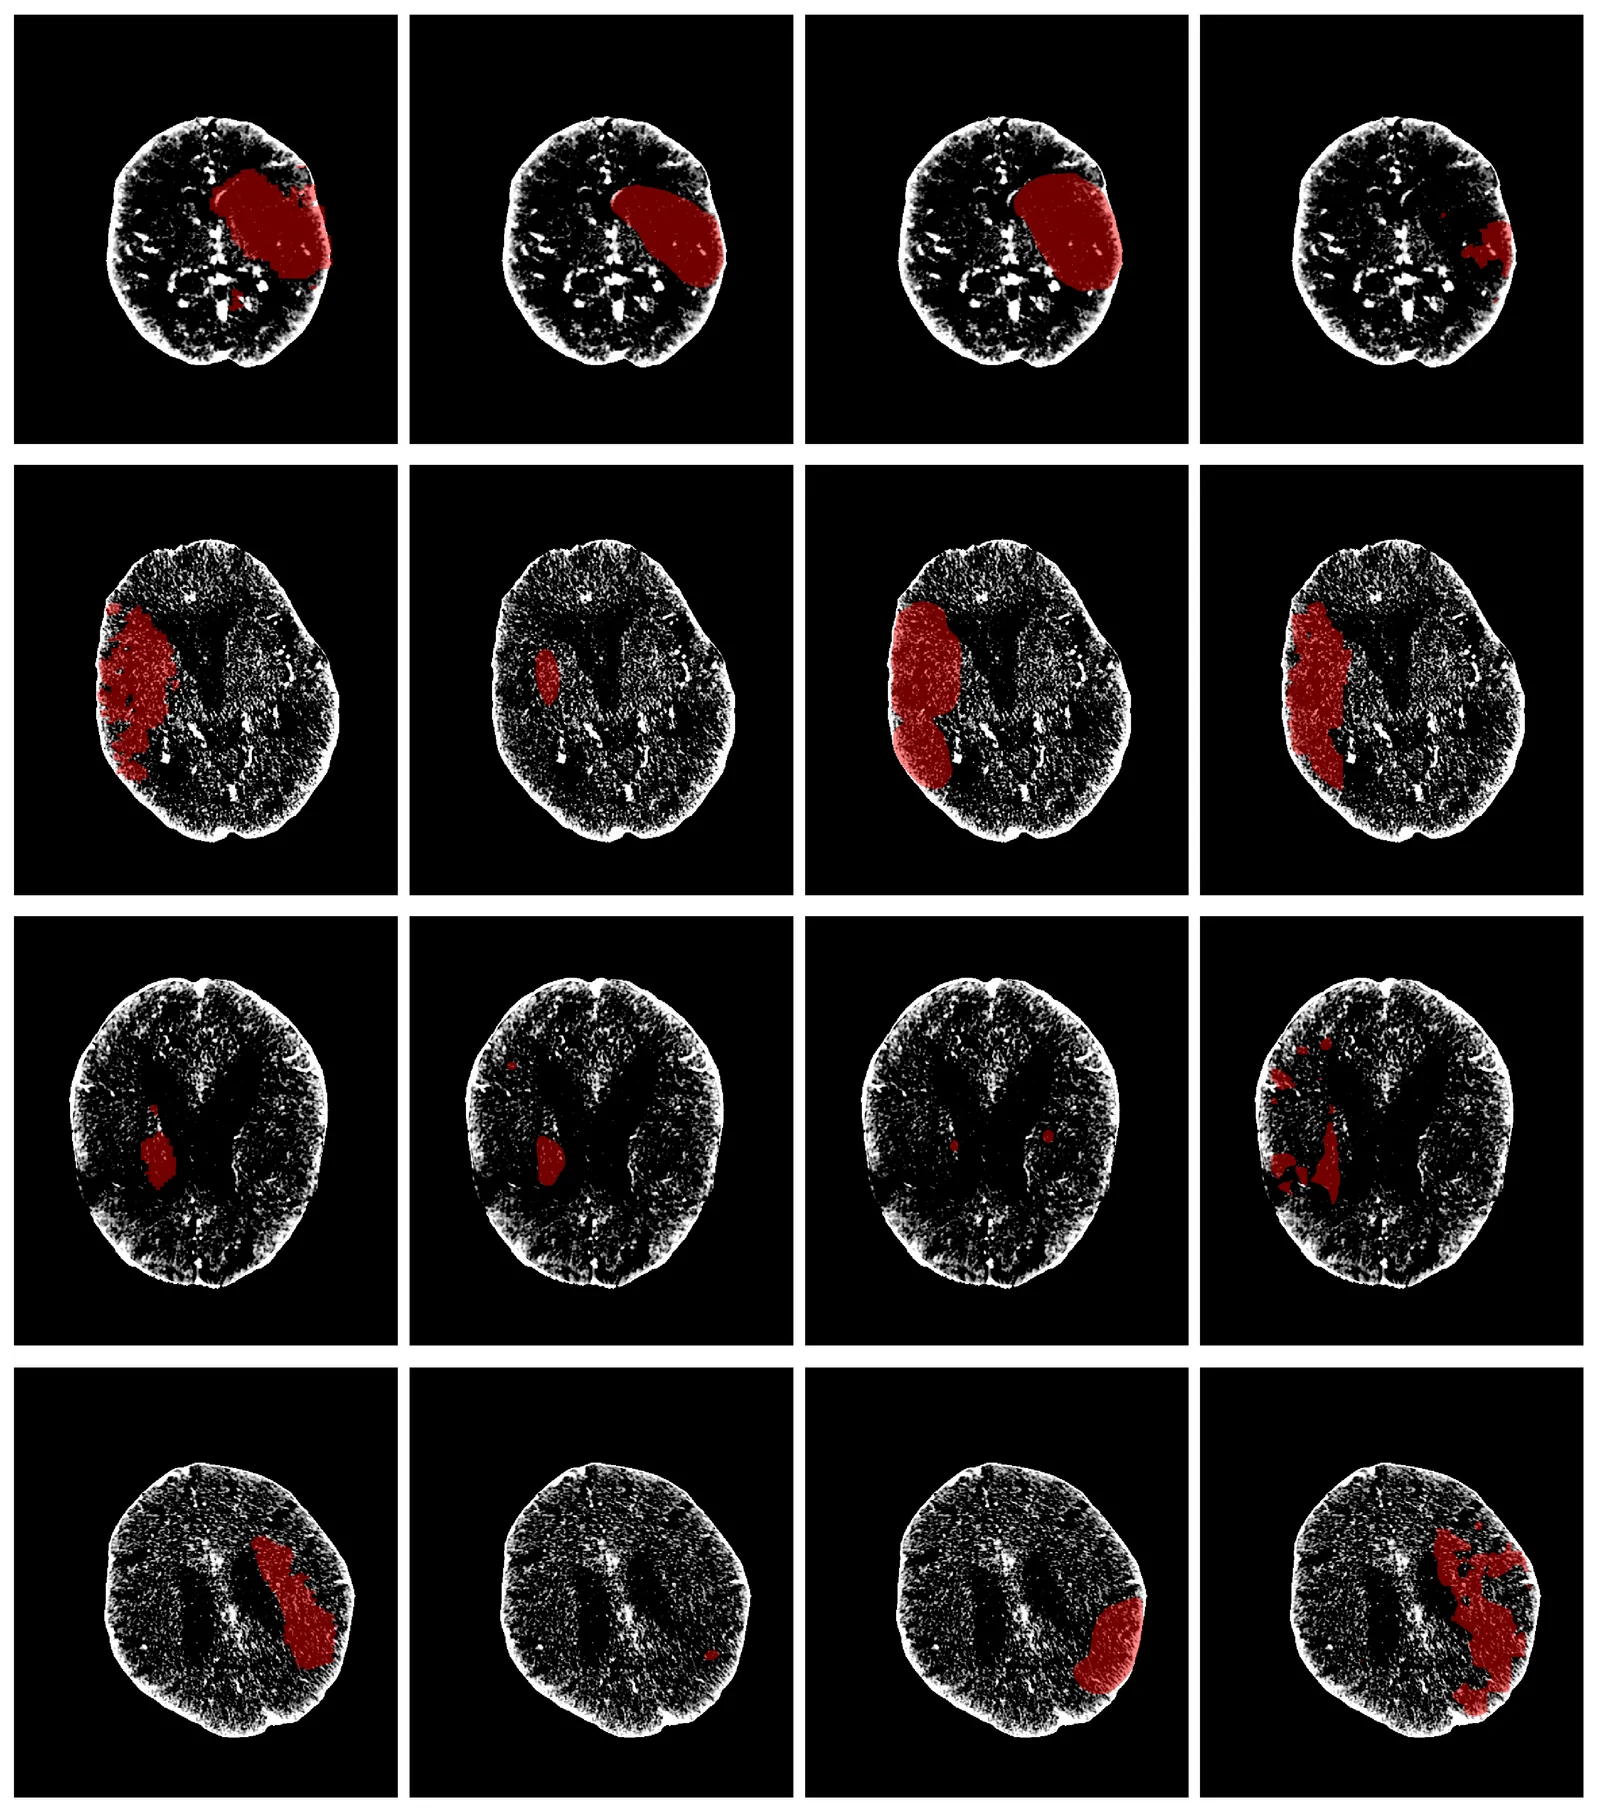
Qualitative comparison of the three segmentation strategies on four representative cases from the held-out test set. The figure is arranged in four rows and four columns. Columns, from left to right: ground-truth infarct mask, M1 prediction, M2.2 prediction, and M3 prediction, all overlaid on the CTA channel as anatomical reference (red region: lesion mask). Row 1 (sub-stroke0027): best CNN case; Transformer underperforms. Row 2 (sub-stroke0082): best Transformer case across the test set. Row 3 (sub-stroke0148): M1 success with simultaneous cascade failure. Row 4 (sub-stroke0007): severe fragmentation across all models.
